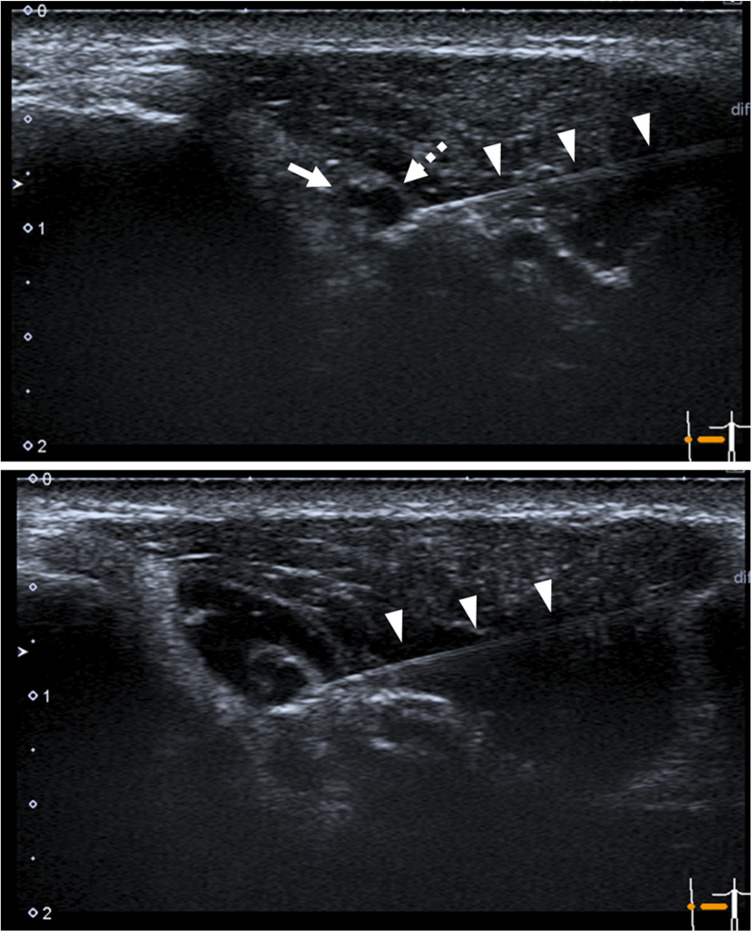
Ultrasound (US) image of US-guided sciatic nerve blocks. Two components of the sciatic nerve, the tibial (*dashed arrow*) and common peroneal nerves (*arrow*), are visualized (*upper panel*). The *arrowheads* indicate the needle trajectory. The injected anesthetic agent is shown as a low echoic area around the tip of the needle (*lower panel*).
